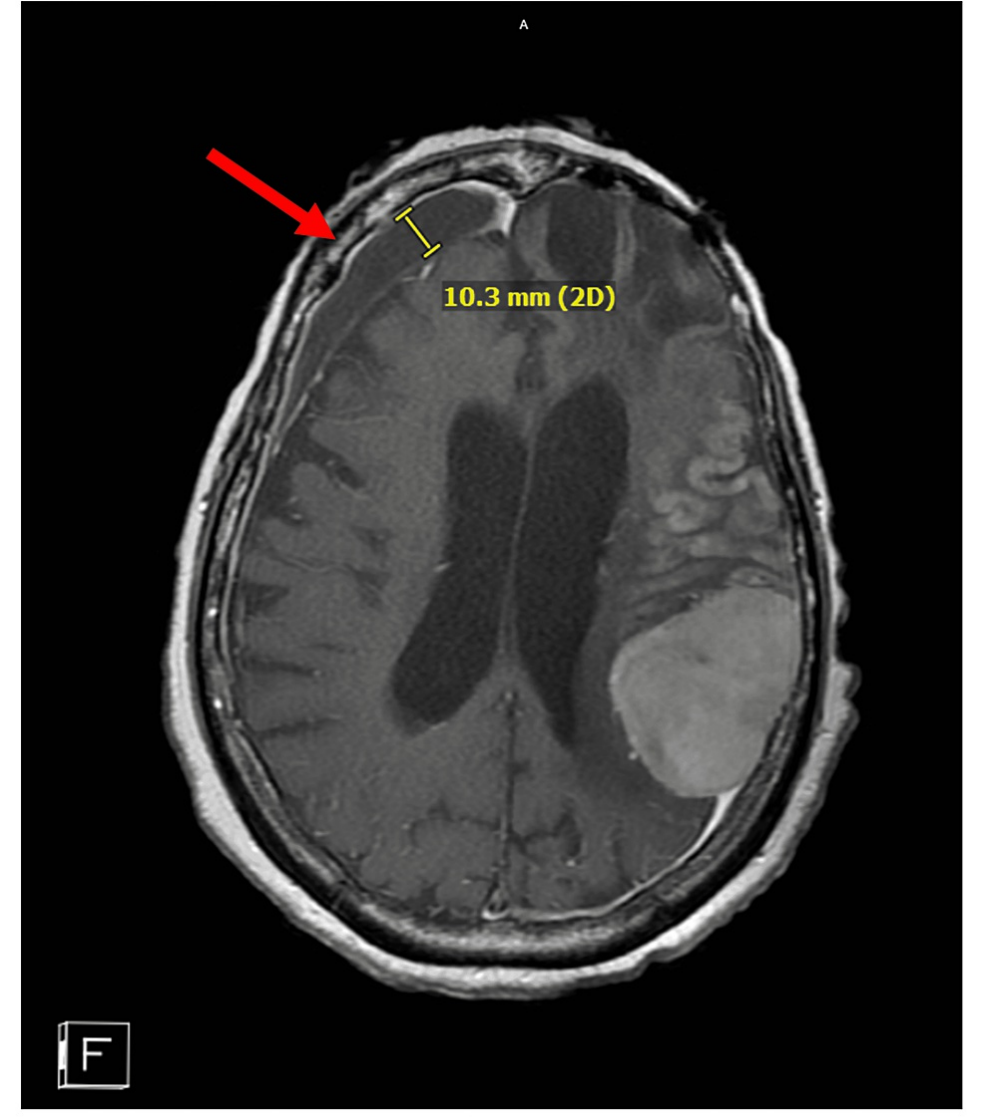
Left frontal subdural hematoma observed in the MRI scan The chronic subdural hematoma (red arrow) measures 10.3 mm as noted by the scale.
